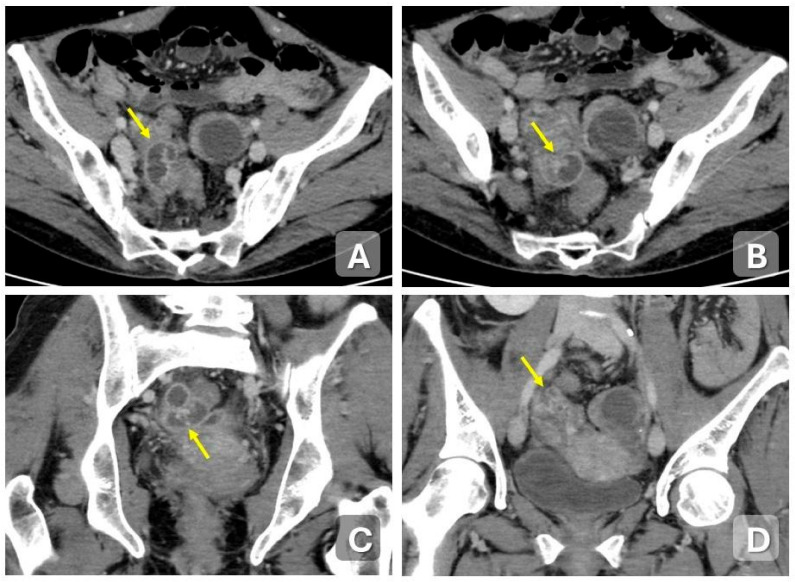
Acute pelvic inflammatory disease: salpingitis in a 27-year-old woman presenting with pelvic pain. Axial (A,B) and coronal (C,D) contrast-enhanced CT images demonstrate a distended fallopian tube with thickened, tortuous walls, exhibiting a characteristic coiled appearance (arrows).
